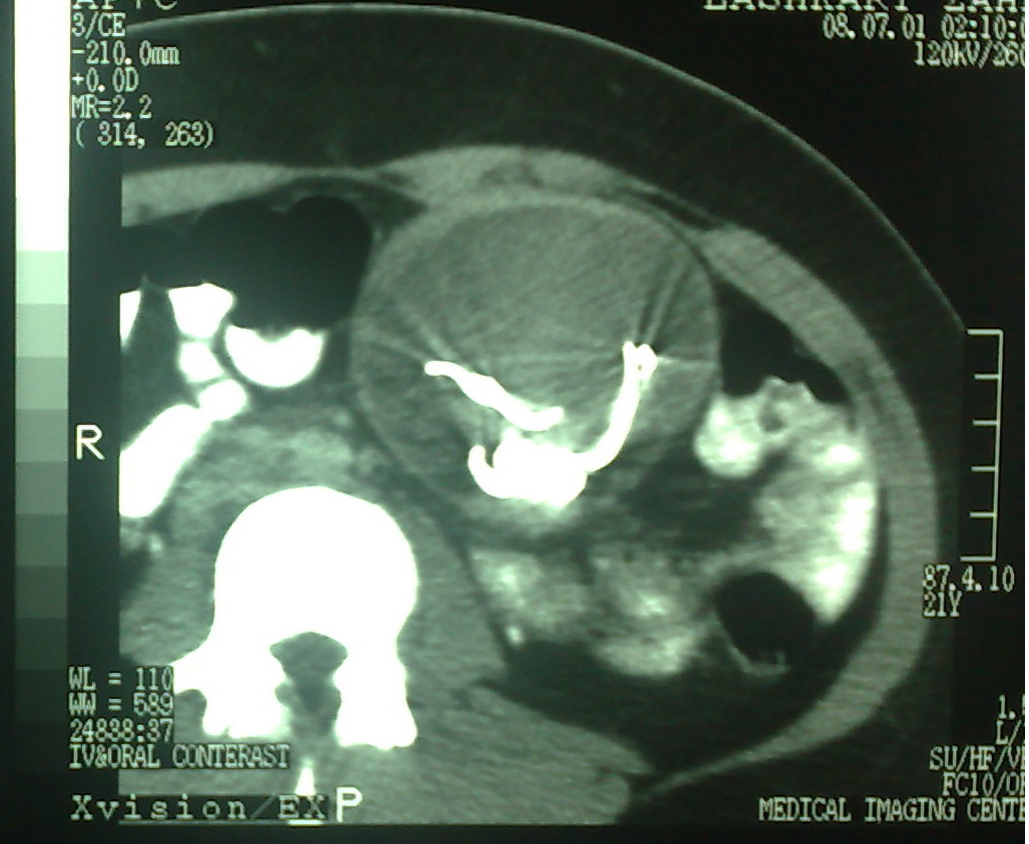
Abdominal CT scan showing a round well-defined soft-tissue mass containing an internal high-density area in the mid-abdomen.
